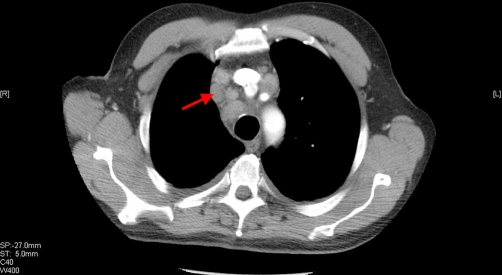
CT scan. Perihilar lymphadenopathy demonstrated on CT in keeping with metastatic carcinoma.
